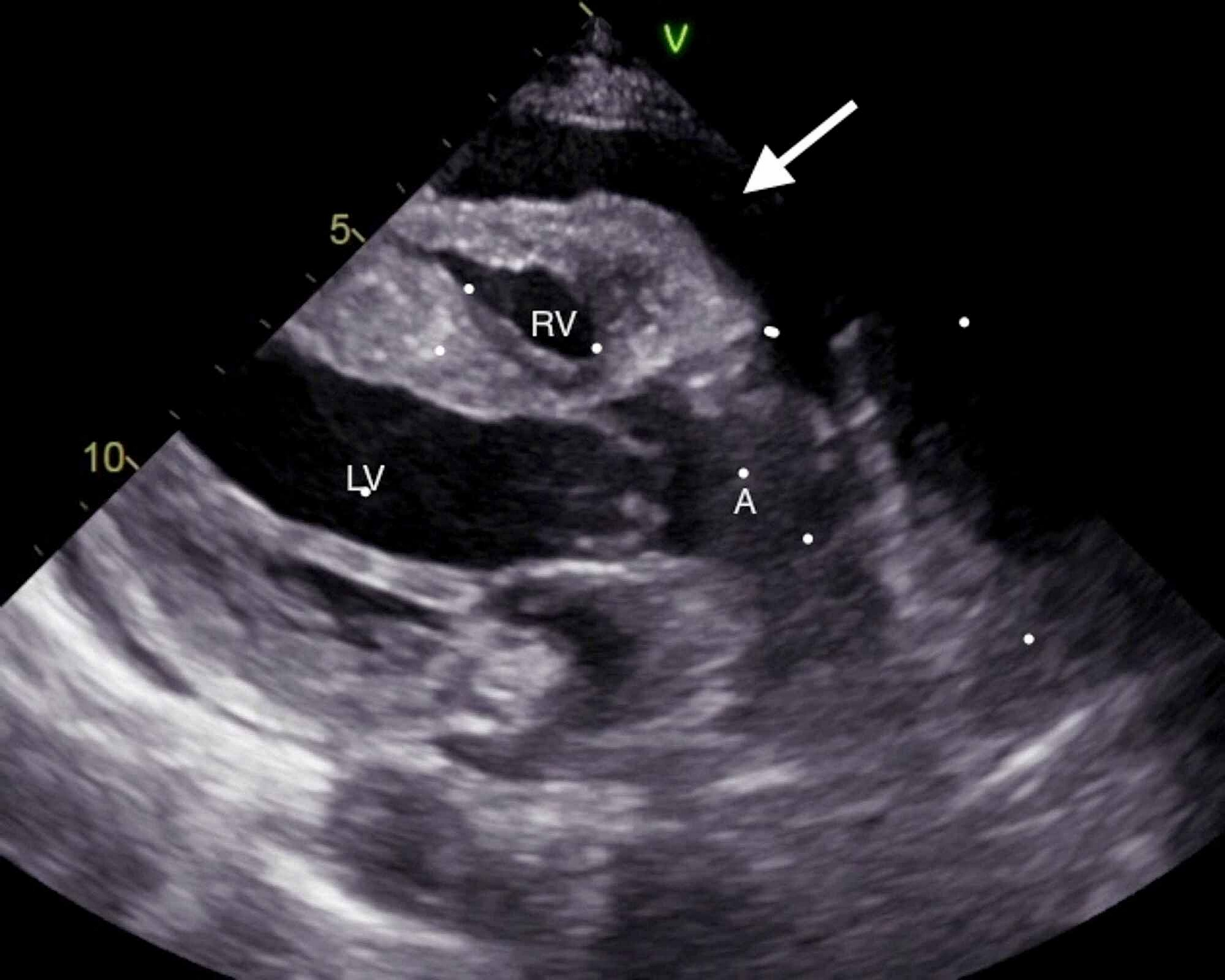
TTE (long para-sternal axis view): anterior pericardial effusion White arrow showing pericardial effusion compressing the right ventricle; TTE: transthoracic echocardiogram; RV: right ventricle, LV: left ventricle; A: aortic root.
